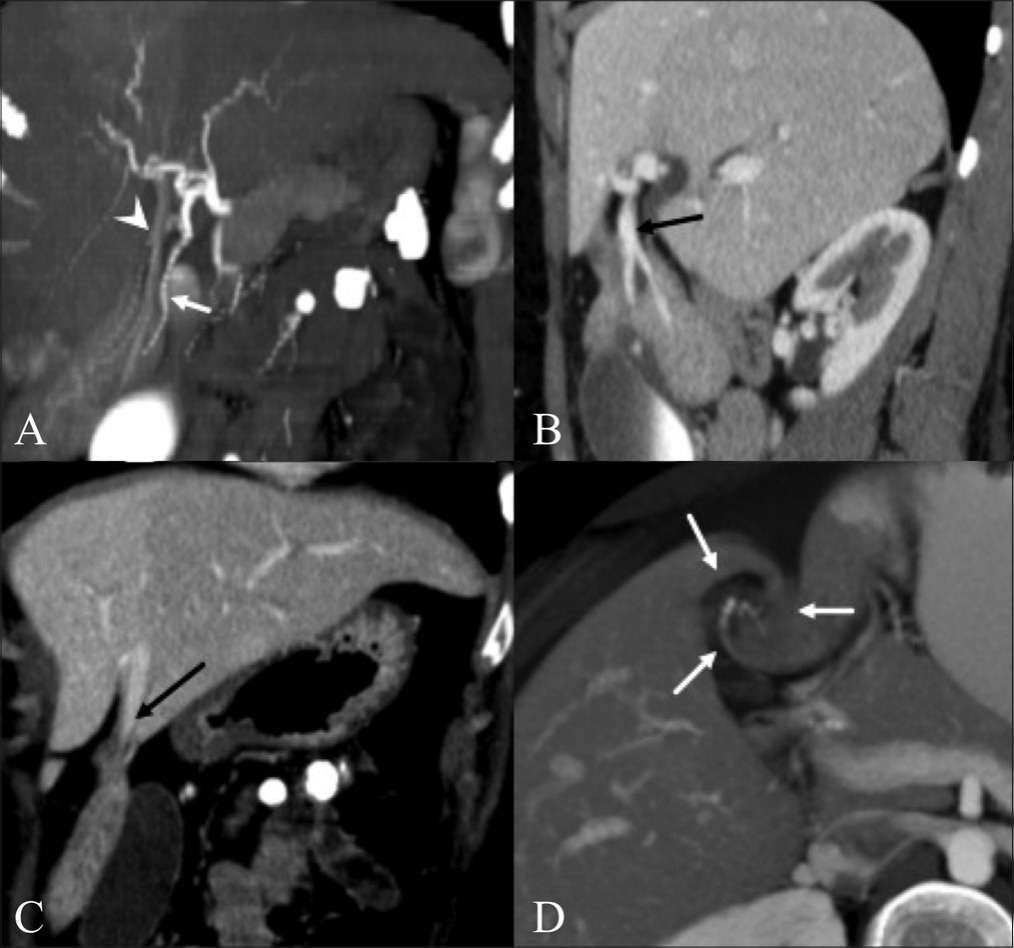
Coronal maximum intensity projection (MIP) arterial-phase CT scan (A) shows anomalous arterial supply (arrow) to the twisted liver segment. The portal venous branches to the accessory lobe are also seen (arrowheads). Sagittal CT scan during the portal venous phase (B) shows an anomalous portal vein branch to the accessory lobe (arrow). An anterior coronal CT scan through the liver (C) demonstrates a branch from the hepatic vein draining the accessory lobe (arrow). Axial thick-slab maximum intensity projection image through the liver (D) demonstrates the twisting of the accessory lobe pedicle (arrows)
